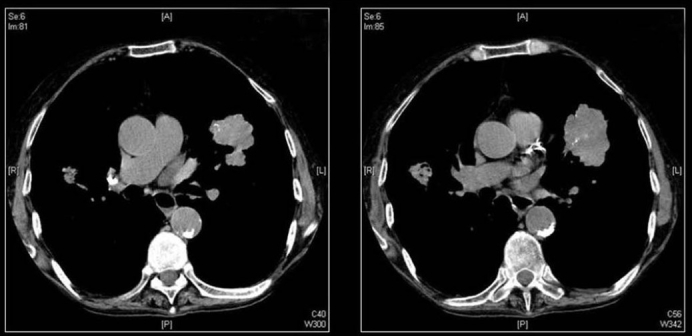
CT scans of the same patient as in Figure 20 shows multiple calcified metastases from a non-mucinous adenocarcinoma from sigmoid colon confirmed on CT guided needle biopsy
